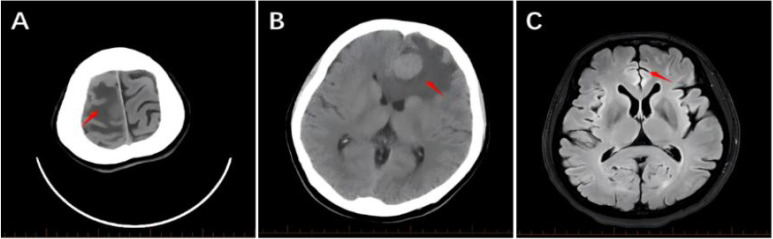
Neuroimaging. (A) Non-contrast head CT obtained on March 3, 2022, shows a hypodense lesion in the right cerebellar hemisphere (red arrow). (B) Follow-up non-contrast head CT on April 7, 2025, reveals a space-occupying lesion in the left frontal lobe (red arrow), measuring approximately 2.7 cm × 2.0 cm with an attenuation value of approximately 33 Hounsfield Units (HU), accompanied by perilesional edema. (C) Brain MRI performed on October 29, 2025, shows significant improvement compared to the study from April 7, 2025 (red arrow).
